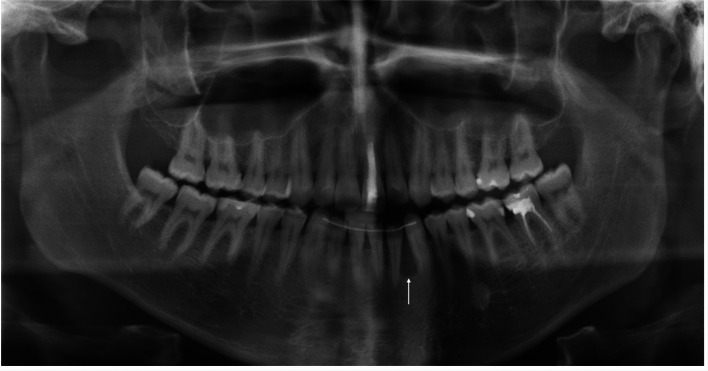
Initial radiological imaging: panoramic radiography exposed circumscribed radiolucency (size 8 × 6 × 5 mm) between 32 and 33 (arrow)
